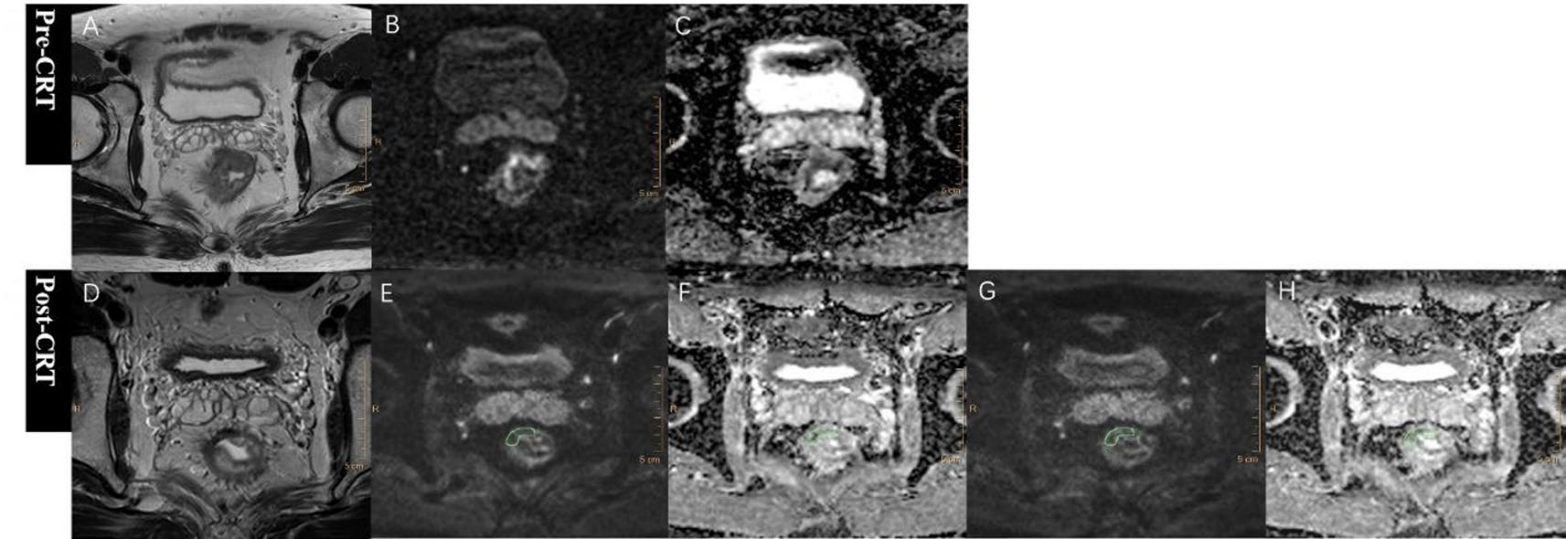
Example of RESOLVE, SMS-RESOLVE DWI and corresponding ADC maps of a 35-year-old male patient, who was diagnosed pathological complete response after surgery. A T2-weighted image before neoadjuvant therapy. B RESOLVE DWI image with b value of 1000s/mm^2^ with ROI delineation on fibrotic rectal wall area at primary tumor position. C Corresponding ADC map of RESOLVE DWI, and the fibrosis area after treatment showed ADC value of 1.689*10^− 3^mm^2^/s. D T2-weighted image after completion of neoadjuvant therapy. E SMS-RESOLVE DWI image with b value of 1000s/mm^2^ with copied ROI delineation from RESOLVE DWI. F Corresponding ADC map of SMS-RESOLVE DWI, and the fibrosis area after treatment showed ADC value of 1.523*10^− 3^mm^2^/s. For clearer visualization of the underlying image anatomy, please see the same cases without ROIs in Supplementary Fig. 2.
